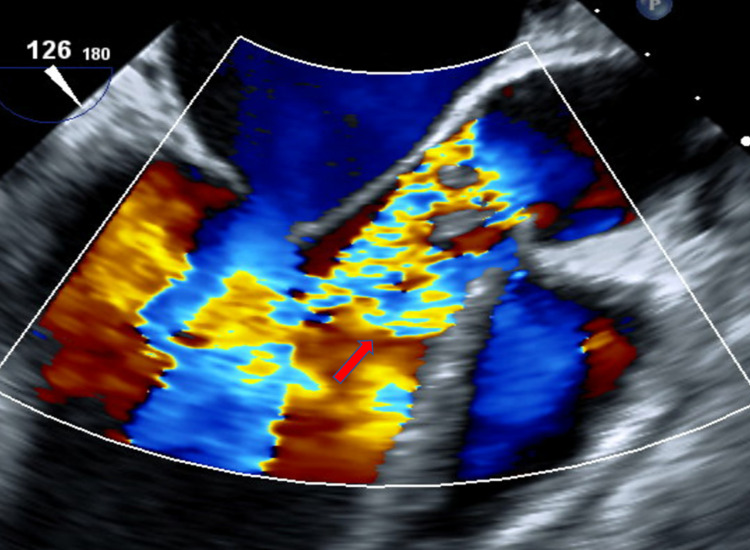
TEE showing severe aortic valve regurgitation (red arrow). TEE, transesophageal echocardiography
